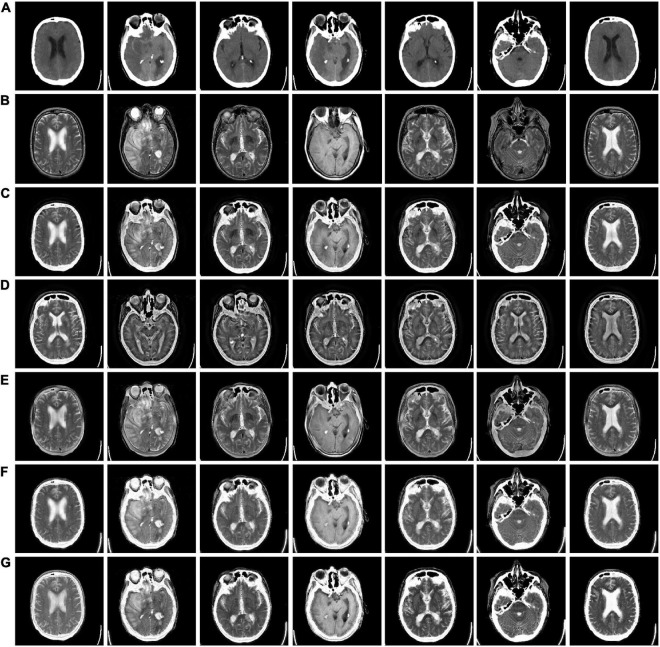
CT-MRI fusion images obtained by five fusion methods. (A) Computed tomography (CT) source image; (B) magnetic resonance imaging (MRI) source image; (C) nonsubsampled contourlet (NSCT) fusion result; (D) non-subsampled shear wave transform (NSST) fusion result; (E) guided filter ng fusion (GFF) fusion result; (F) Laplacian redecomposition (ReLP) fusion result; (G) fusion result of the proposed method.
